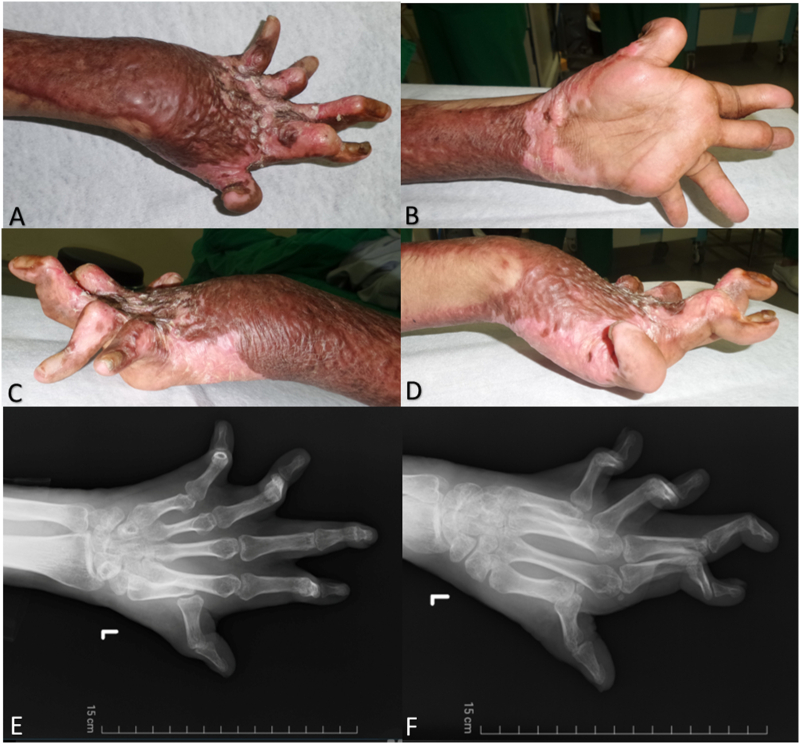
Clinical picture of the upper left extremity of the patient at first presentation. The left dorsal hand had extensive scars, forming swan neck deformity on the middle finger and Boutonnières deformities on the other fingers. All five digits were hyperextended at the MCP joint. The picture at the dorsal view (A), palmar view (B), lateral ulnar view (C), lateral radial view (D), and X-ray (E-F).
